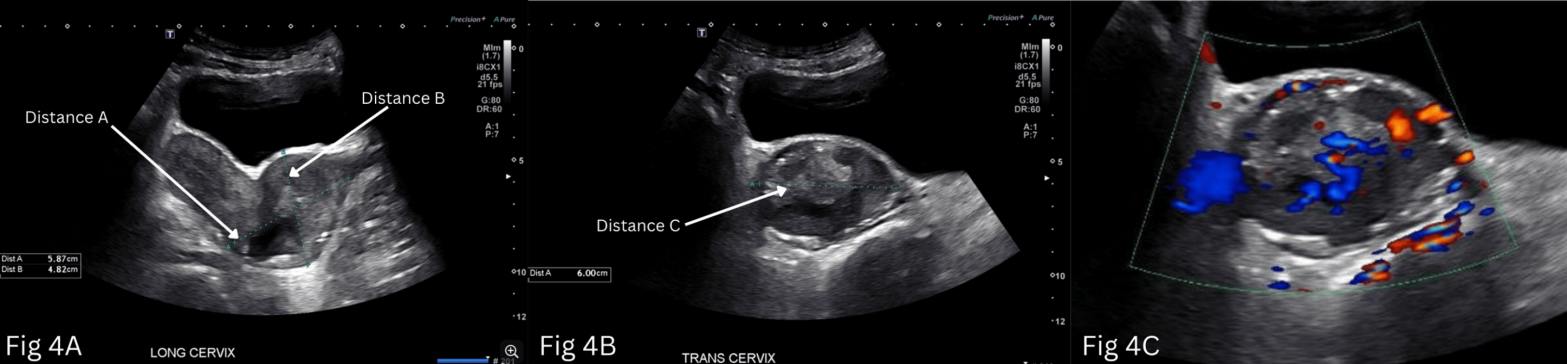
Transabdominal ultrasound in B mode showing longitudinal view (A) and transverse view (B) of the uterus. C. Transabdominal ultrasound of uterus in Doppler mode. (A) Longitudinal view: Distance A represents the length of 5.9 cm, Distance B represents width of 4.8 cm. (B) Transverse view: Distance C represents the height of 6 cm. Transabdominal ultrasound of uterus in Doppler mode (C) demonstrating areas of vascularity.
